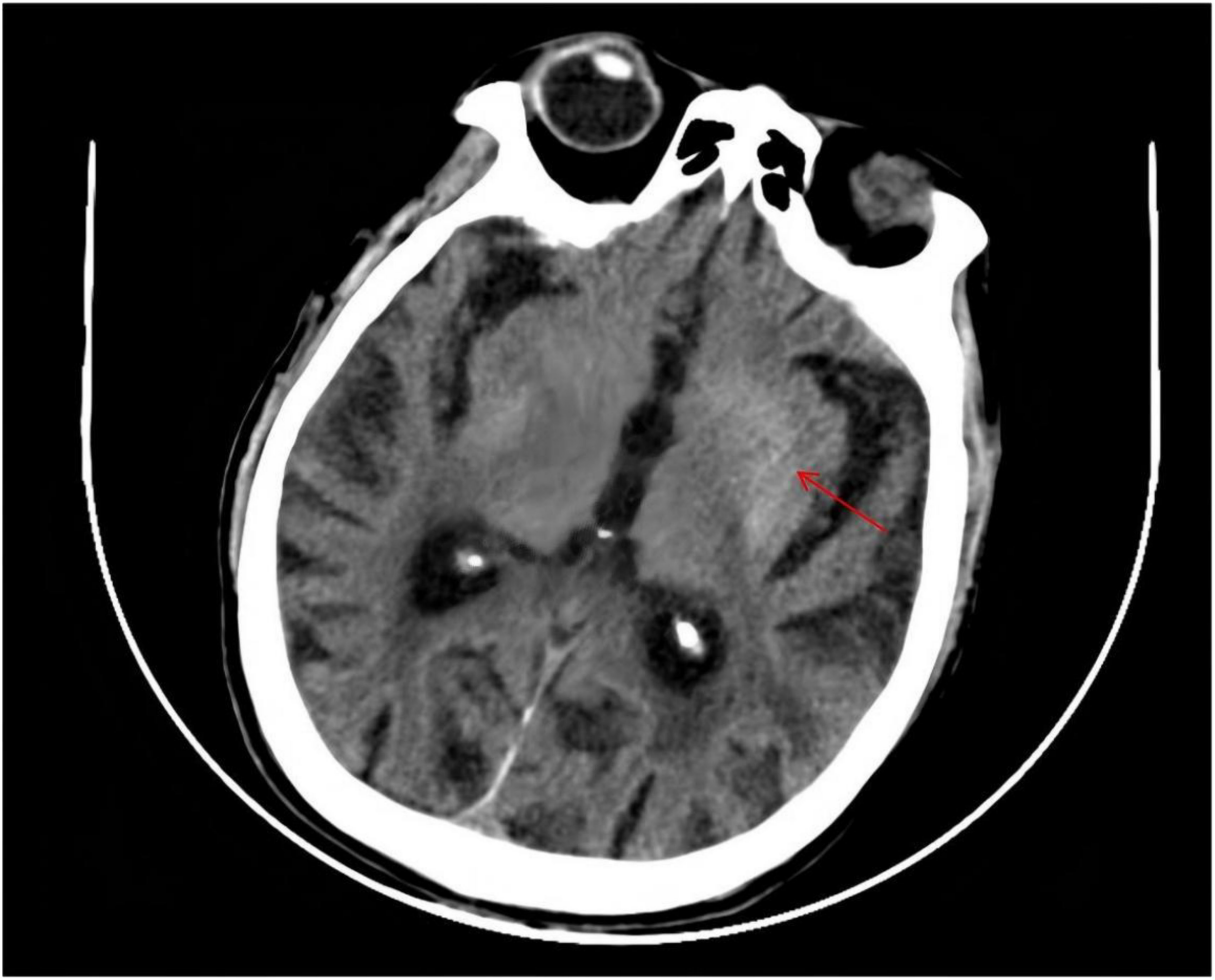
The CT scan reveals slightly low-density areas with a striped or linear configuration in the left parieto-occipital lobe, measuring approximately 3.0×3.6 cm.The CT value of the abnormal lesion center is approximately 36 HU. Abnormal signals were marked by red arrow.
